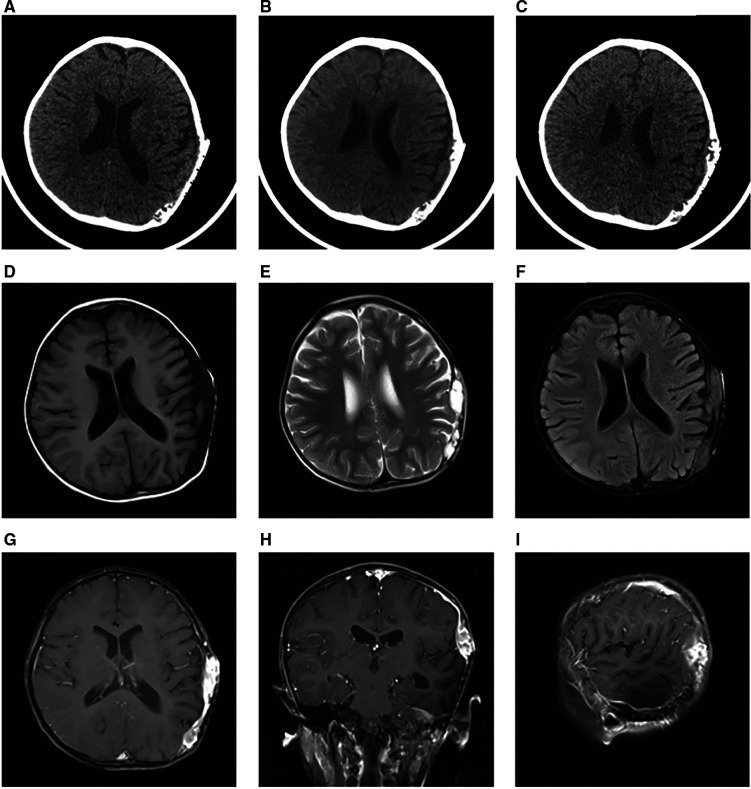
One year after the first operation, the CT scan showed multiple cystic bone destructions around the original cranial repair site (A–C). Around the repaired skull, the tumor revealed long signal abnormal signals on T1-weighted and T2-weighted images (D,E). In FLAIR, it was an iso-low signal (F). Contrast-enhanced MRI images also showed a markedly enhanced mass (E–G).
